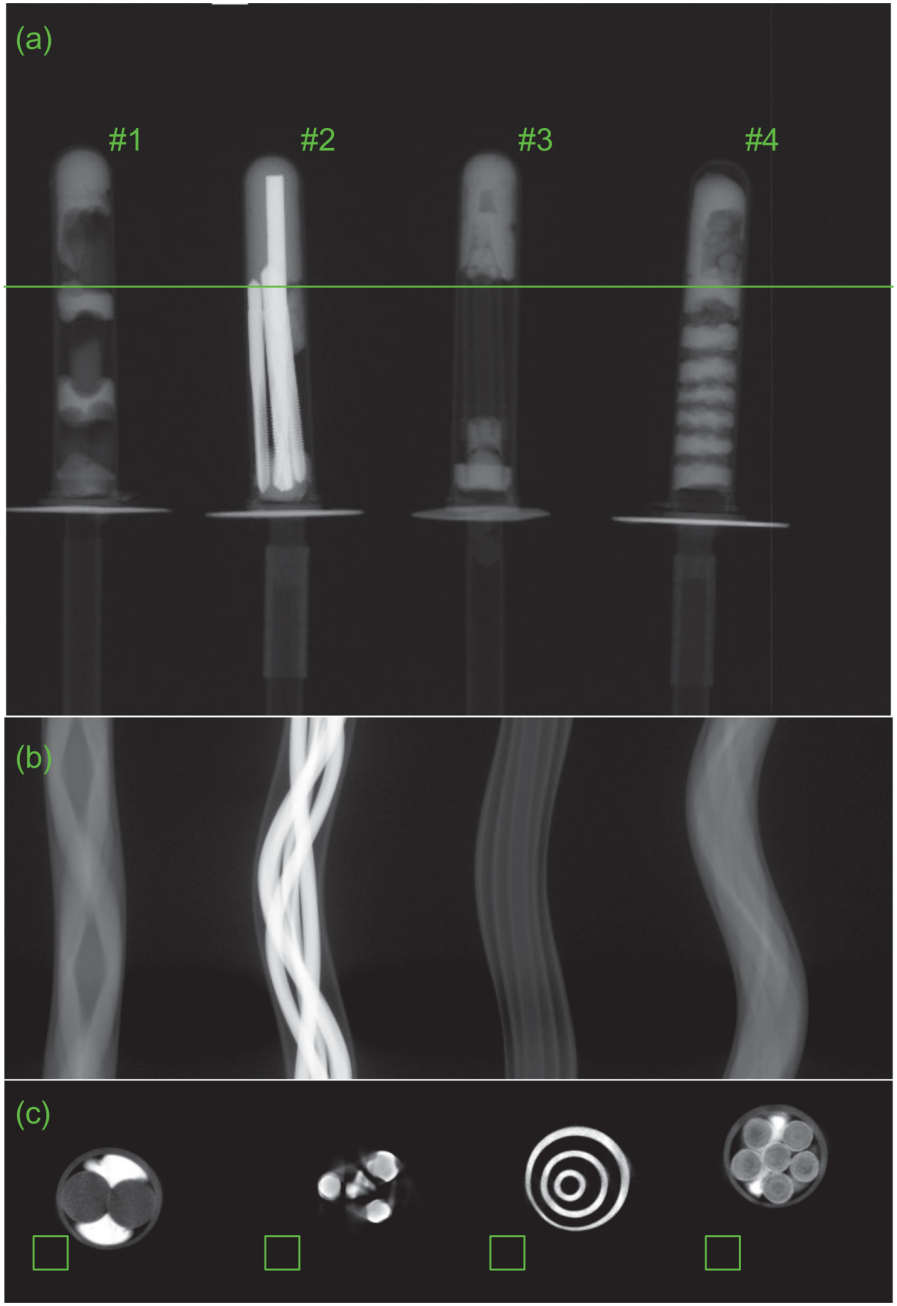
The multi-mounted CT experimental results. (a) is one of the two-dimensional projection images, (b) the projection sinogram and (c) the reconstructed images with ART in Eq (1).
